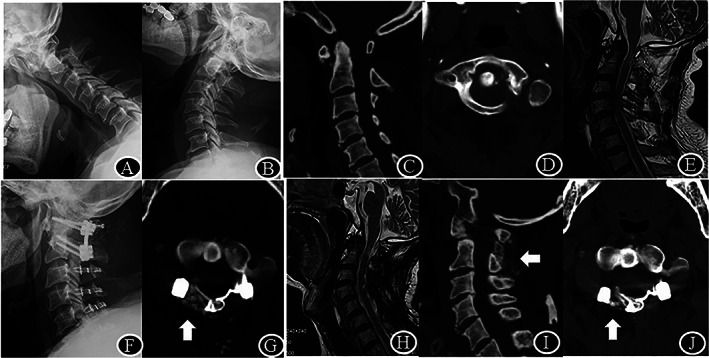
The imaging of patient no. 3, male, diagnosed as atlas hypoplasia and subaxial cervical spinal stenosis. (A–D), ISD of 29 mm, SAC of 8 mm and ADI of 4 mm pre‐operation; (E), Preoperation MRI shows posterior compression at the level of atlas and C3/4, C4/5; (F), Postoperative X‐ray of the same patient shows C1‐2 pedicle screws and C1, C3‐6 laminar mini‐plate; (G), ISD of 36 mm, SAC of 23 mm and ADI of 1.5 mm on axial‐CT immediately post‐operation and autogenous iliac bone graft; (H), Sagittal T2–weighted image shows full posterior decompression at 25 months after laminoplasty; (I, J), Sagittal and axial CT identified the healing of the hinge fracture and successful interlaminar fusion
